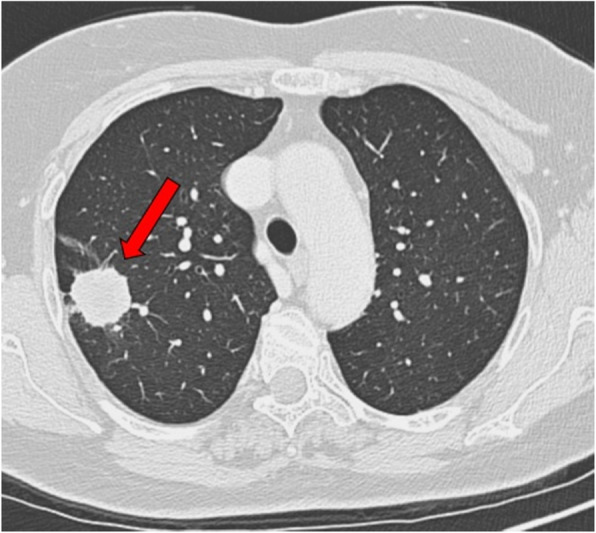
CT scan of chest performed in January 2019 to exclude pulmonary embolism, showing a mass of 28 mm in the right upper lobe (case report 2)
